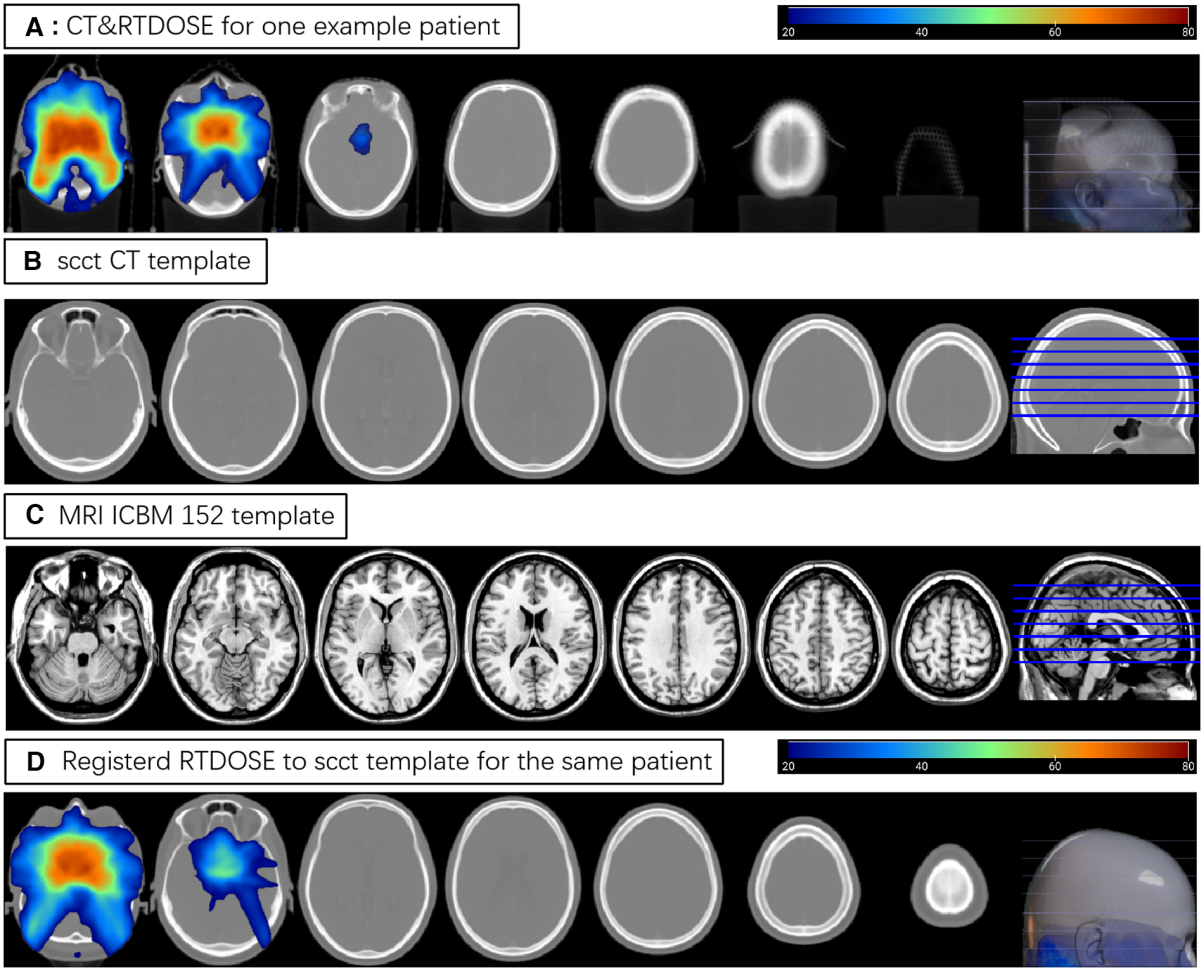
(A) shows some slices of the CT and RTdose images for one example patient; (B) shows slices of the CT template created by Christopher Rorden; (C) shows slices of the MRI T1 template in MNI space created by ICBM; (D) shows slices of the RTdose images registered to the CT template created by Christopher Rorden for the same patient.
